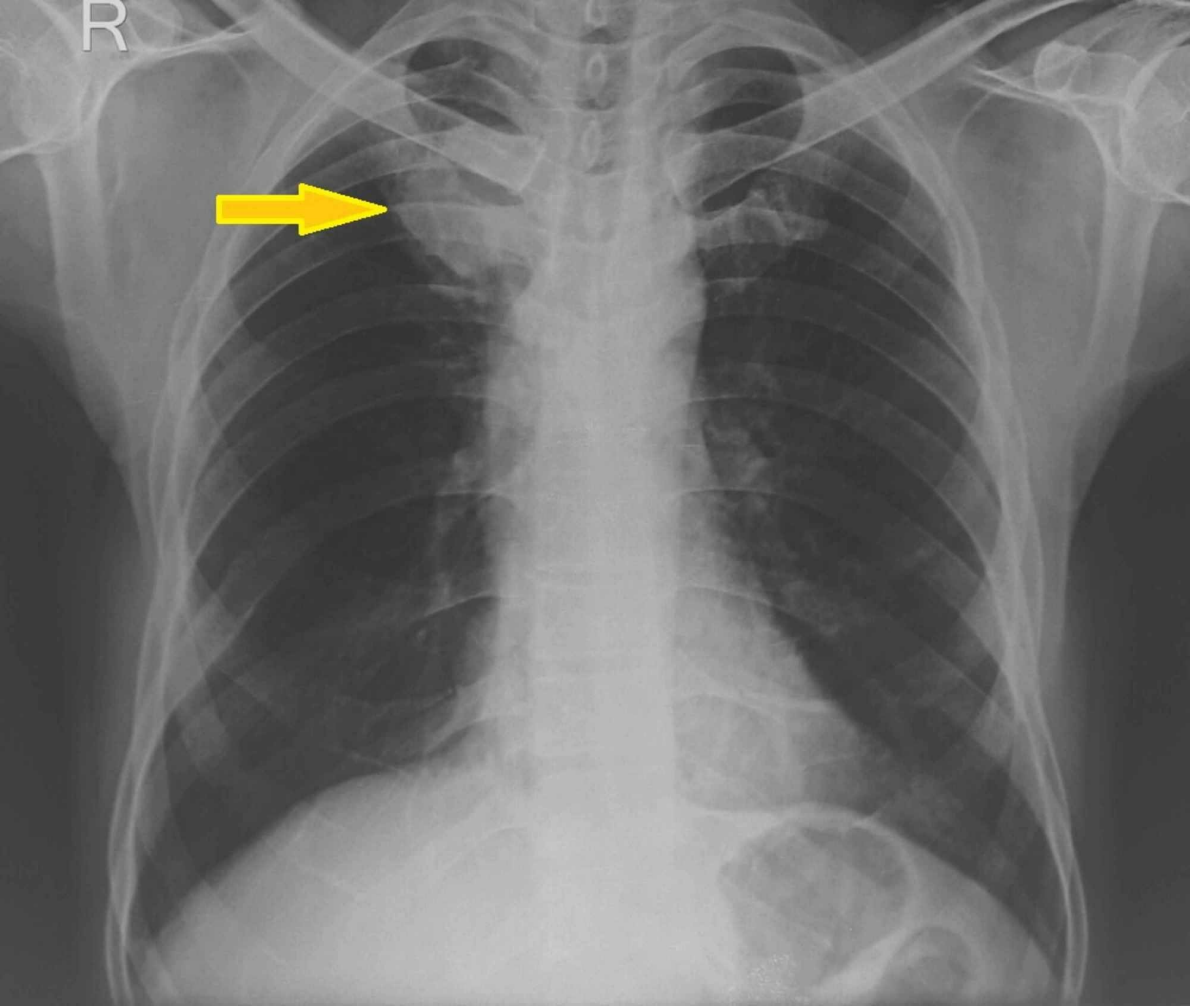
Chest X-ray showing NSCLC lesion causing SVC syndrome. Note a poorly defined soft tissue mass in the right upper zone [9].
